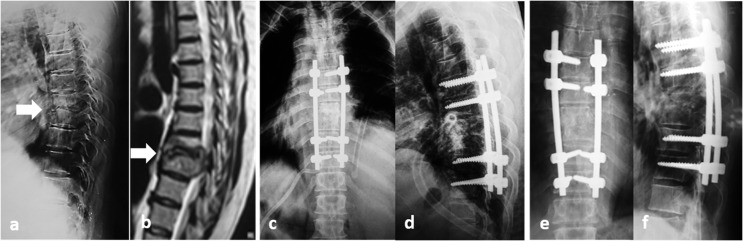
SD D8–9 in a 58-year-old female. (a and b) Preoperative lateral X-rays and MRI sagittal veiws. (c and d) Immediate postoperative X-rays showing partial corpectomy D8–9 with interbody fusion using bone garft and long segment pedicular screw fixation from D6–11 (Posterior approach). (e and f) Follow-up X-rays after 6 months.
